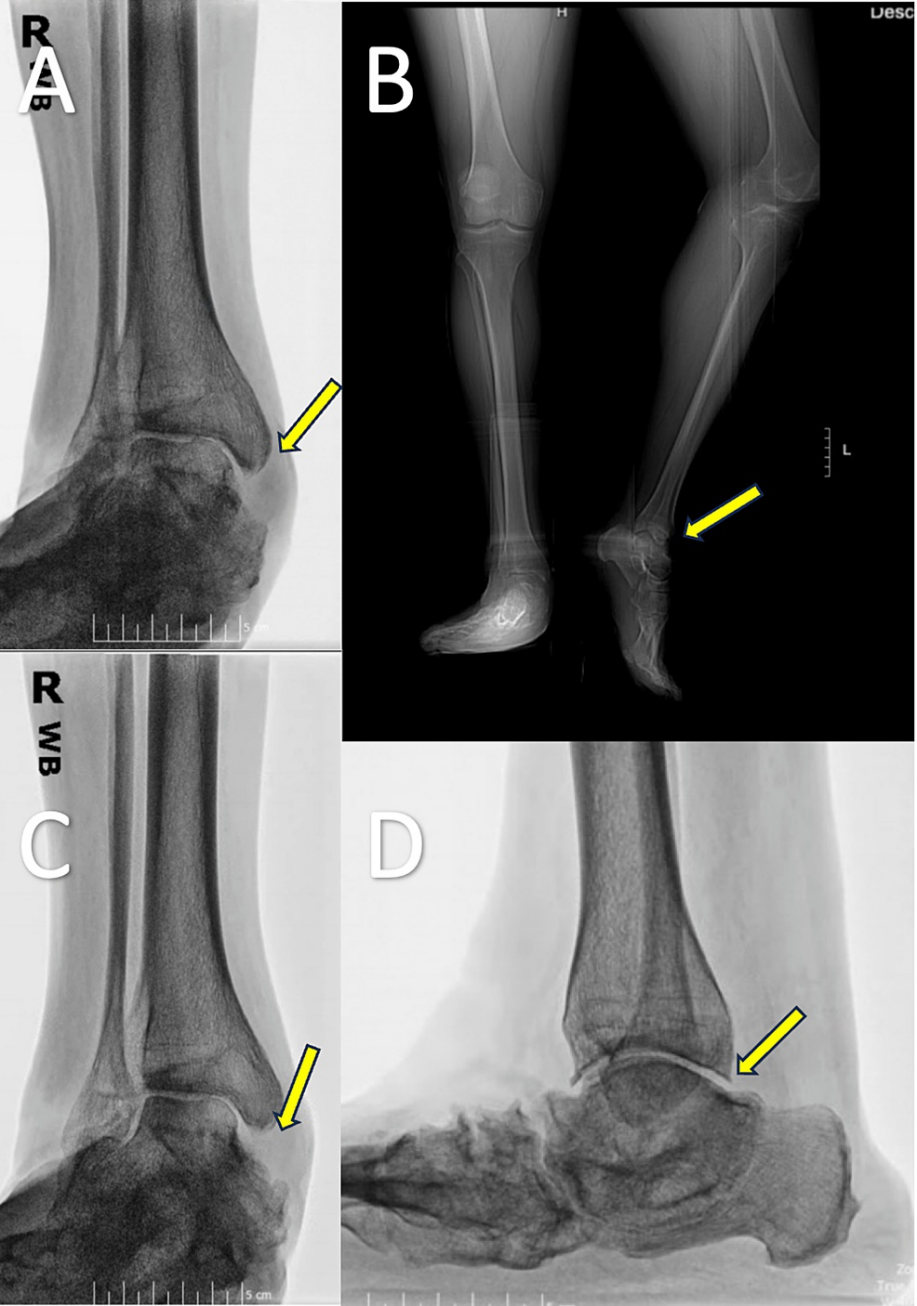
Radiographs of the patient’s left ankle during initial consultation. (A) Anterior-posterior X-ray of the right ankle, showing extensive valgus deformity. (B) Posterior-anterior X-ray of the right ankle, with significant plantar flexion of the ankle. (C) Posterior-anterior X-ray of the right ankle, showing extensive valgus deformity. (D) Mediolateral view X-ray of the right ankle, with significant subtalar joint space narrowing.
